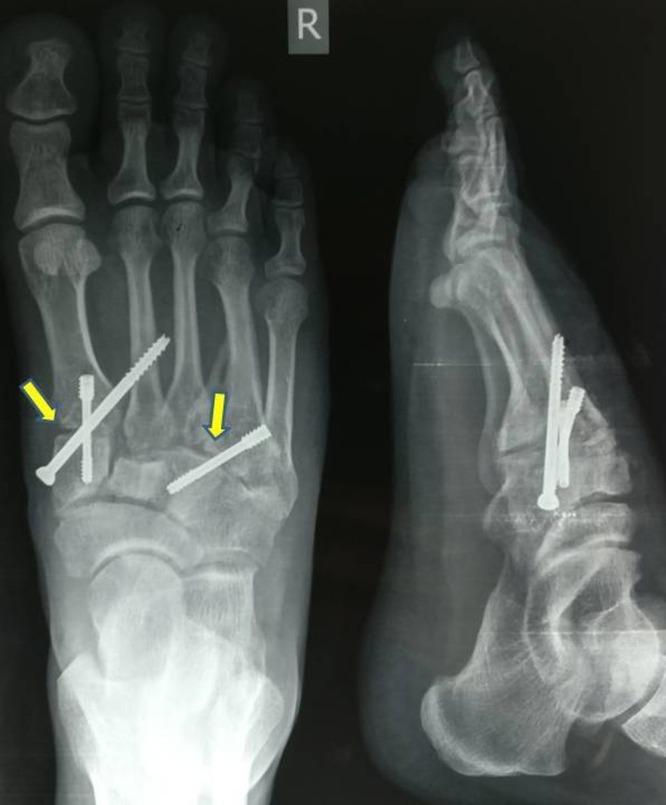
Post-op radiograph (antero-posterior and lateral views) Shows fixation of first, second, and fourth TMT joints with screws and presence of bone graft.
